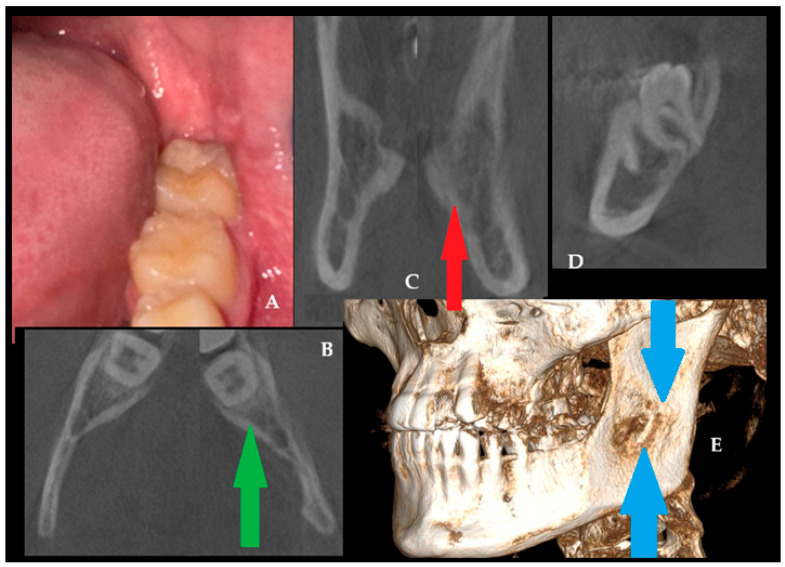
Healing results after a 24-month time frame (A,B). Bone swelling has disappeared ((C), red arrow), while the bone structure behind the second mandibular left molar is well restored (B,D), green arrow). Three-dimensional CBCT evaluation (blue arrows) demonstrates satisfactory bone healing and restoration of normal bone structure (E).
